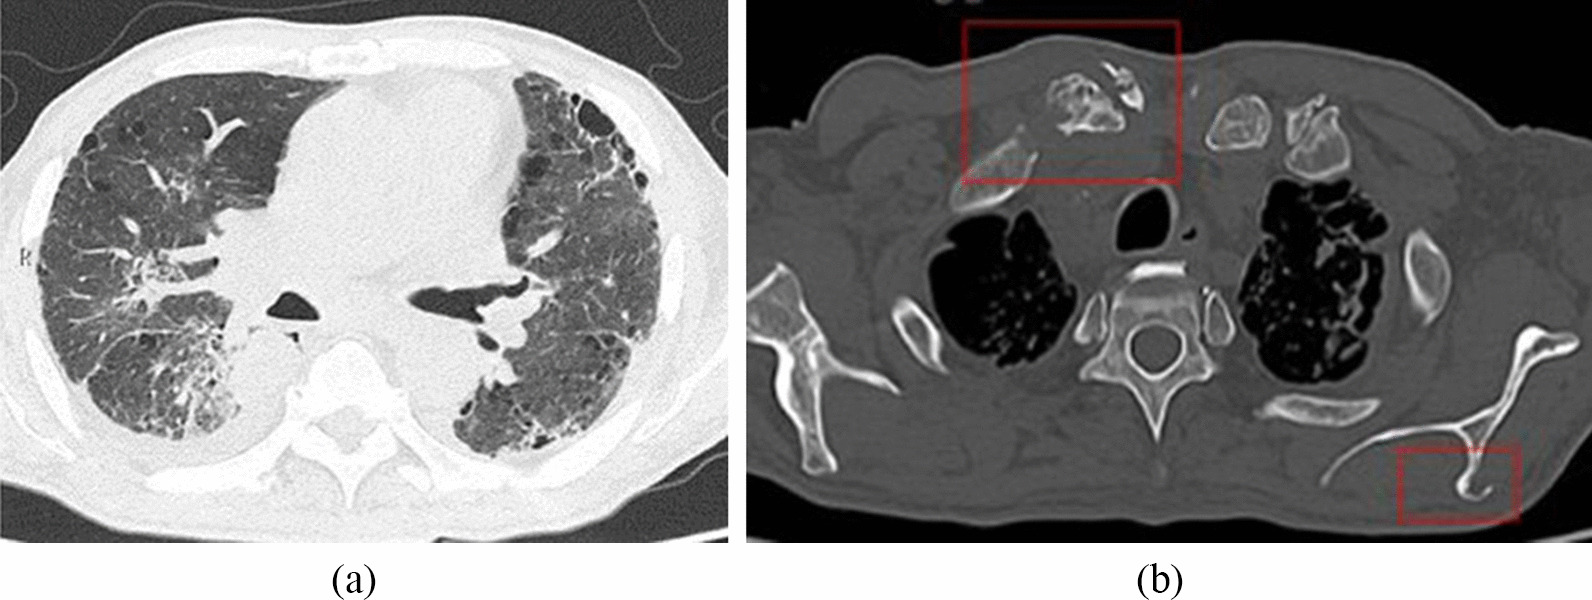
Chest computed tomography scans showed a mass in the right S6 lung region. Pleural effusion and emphysematous changes were also observed (a). Osteolytic changes were observed in the right clavicle and left scapula (b)
